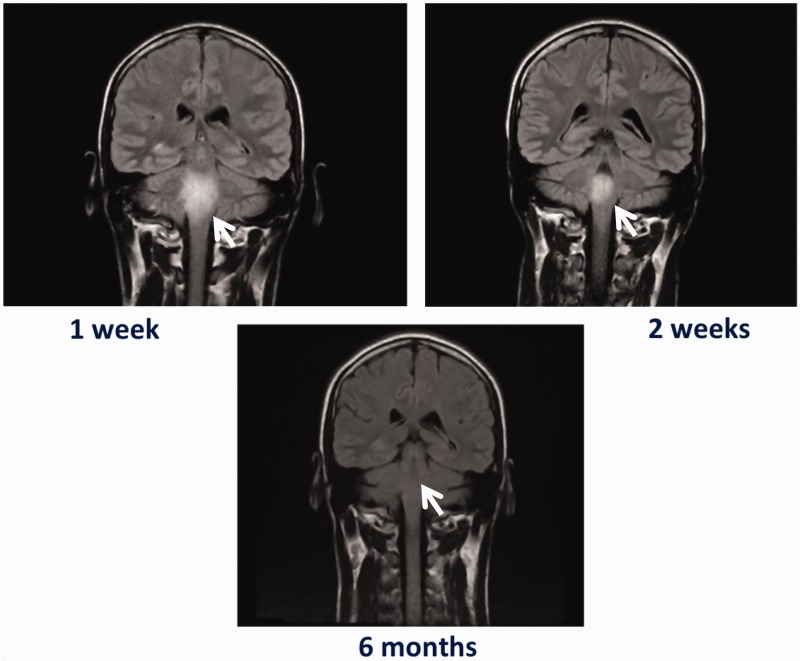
Staged T2-weighted MRI scans showing a significant interval improvement in the intracranial appearance, with almost a complete resolution of the signal abnormality.
